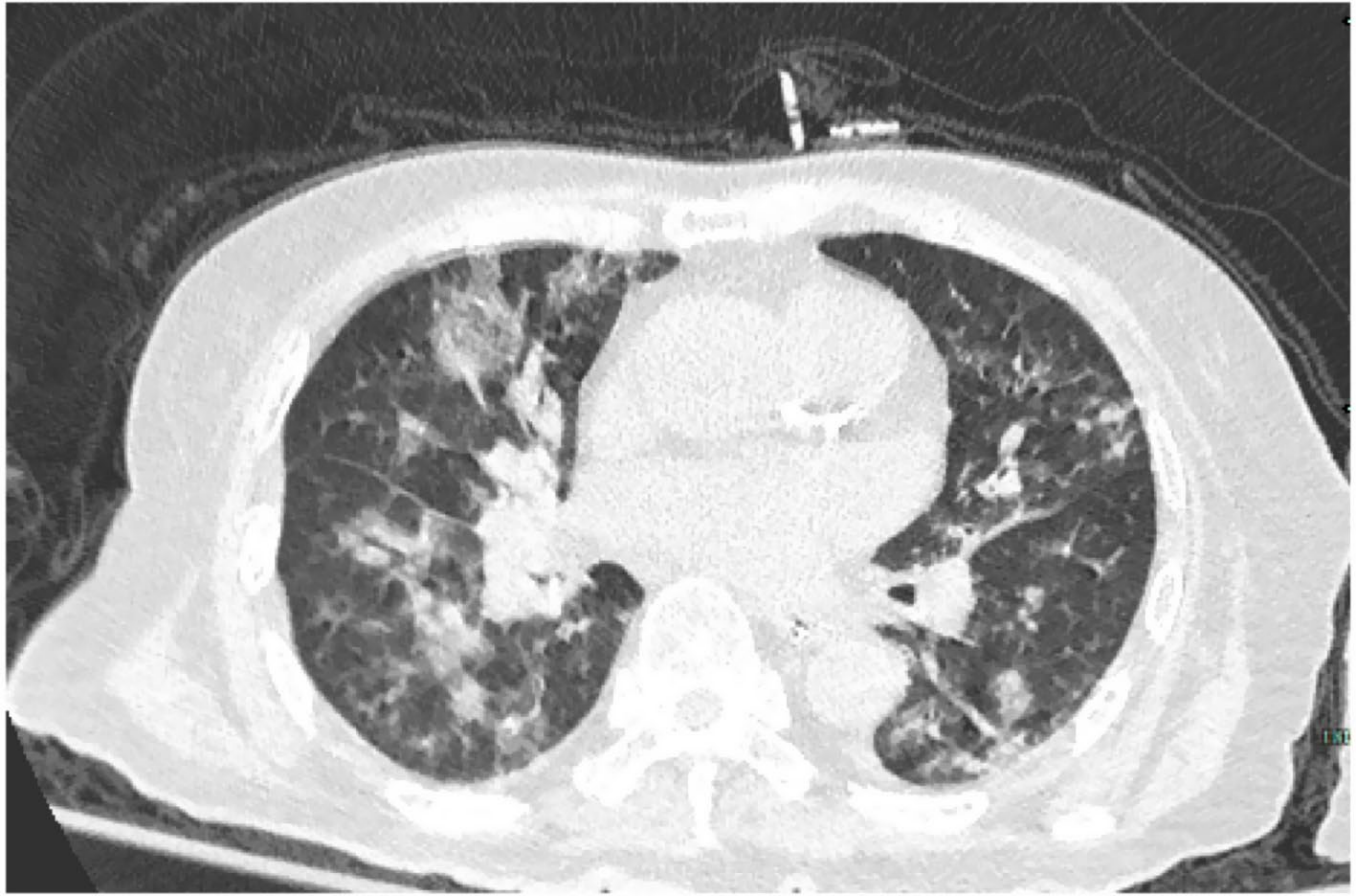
The chest CT scan showed patchy ground-glass lesions in the lungs, mainly along the bronchi.
